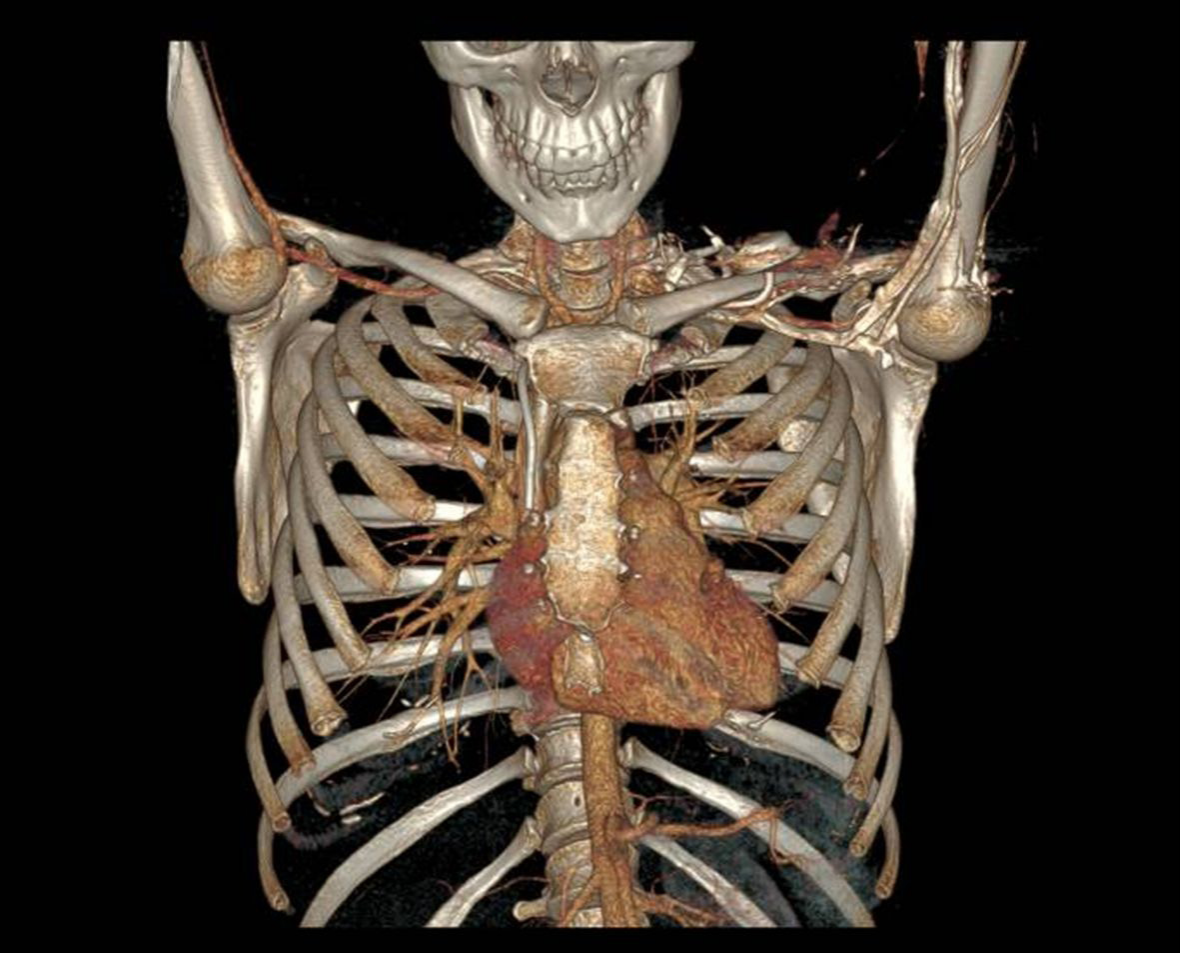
Three-dimensional computed tomography reconstruction also showing the catheter positioned in the right internal thoracic vein.
